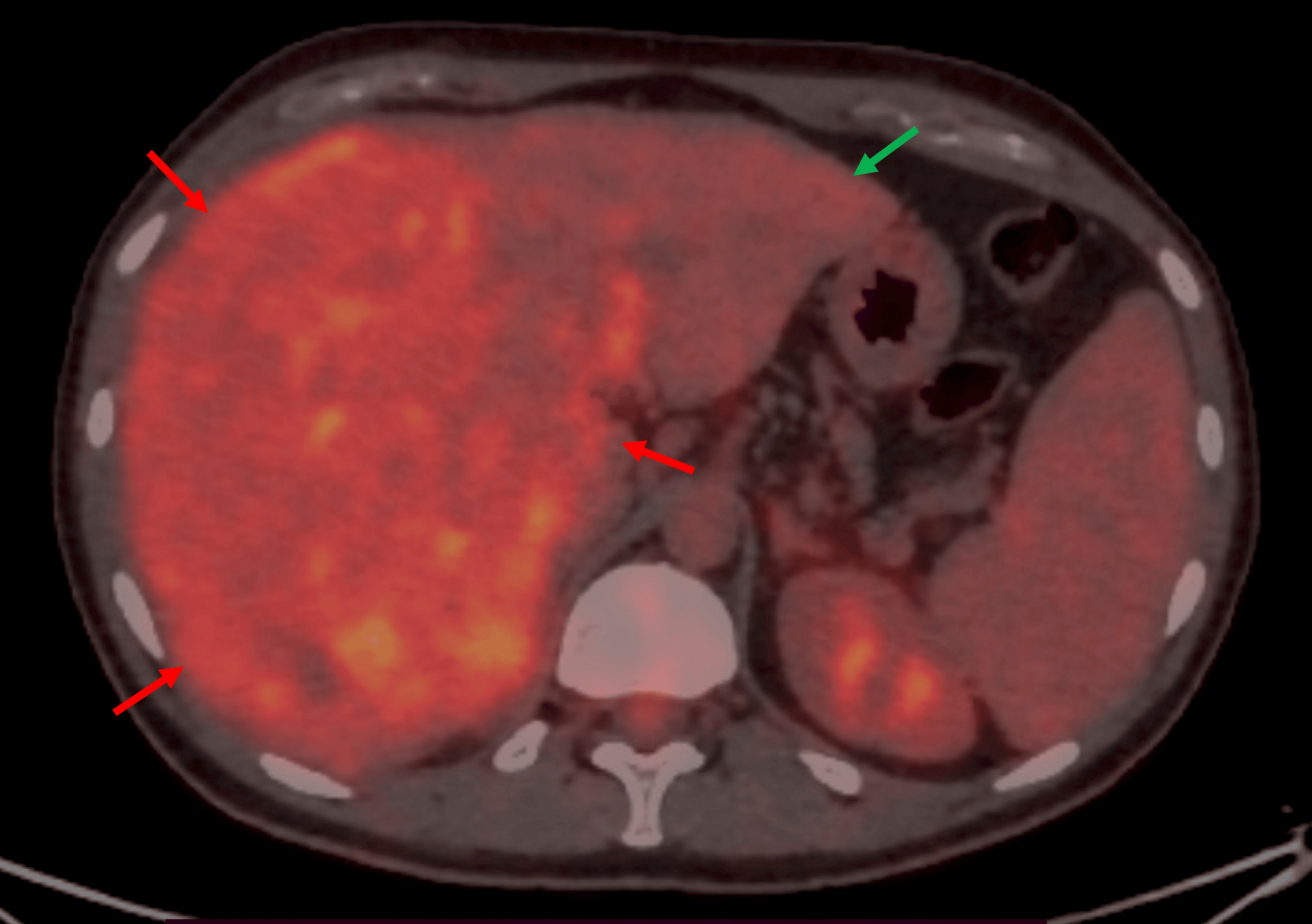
MTM-HCC findings on PET-CT scan Intense, diffuse, and inhomogeneous FDG uptake is observed in the hepatic tumor mass. The tumor is marked by red arrows, and the preserved left lobe is indicated by a green arrow. MTM-HCC: macrotrabecular-massive hepatocellular carcinoma, PET-CT: positron emission tomography–computed tomography, FDG: fludeoxyglucose-18
